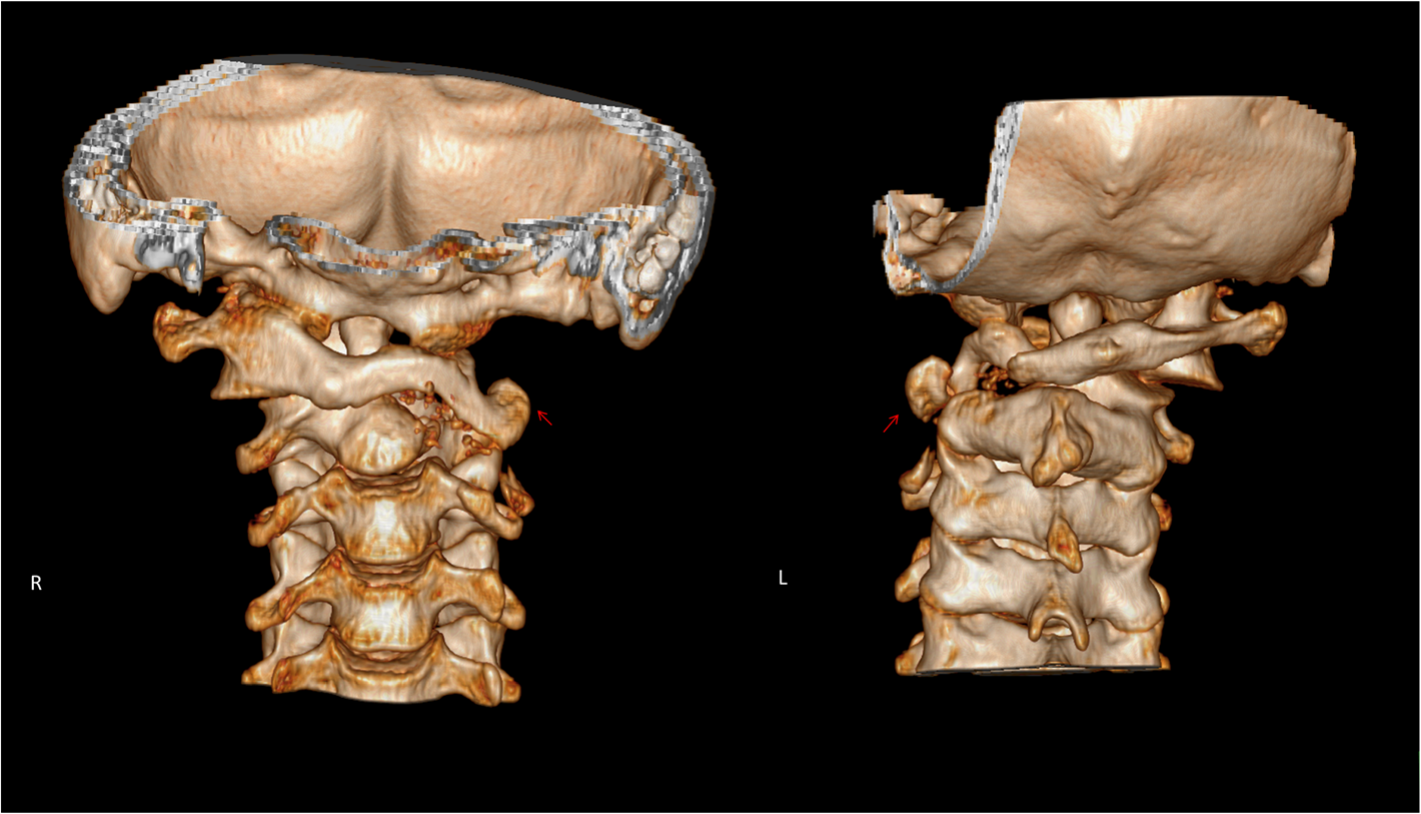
Preoperative 3D-CT examination revealed left lateral mass destruction of Atlas caused by tuberculosis lesion and the loss of atlantoaxial stability
